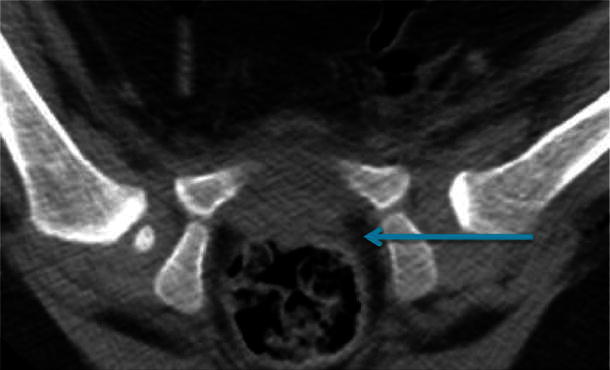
Postoperative single slice CT showing the posterior neck line in a reduced left hip
